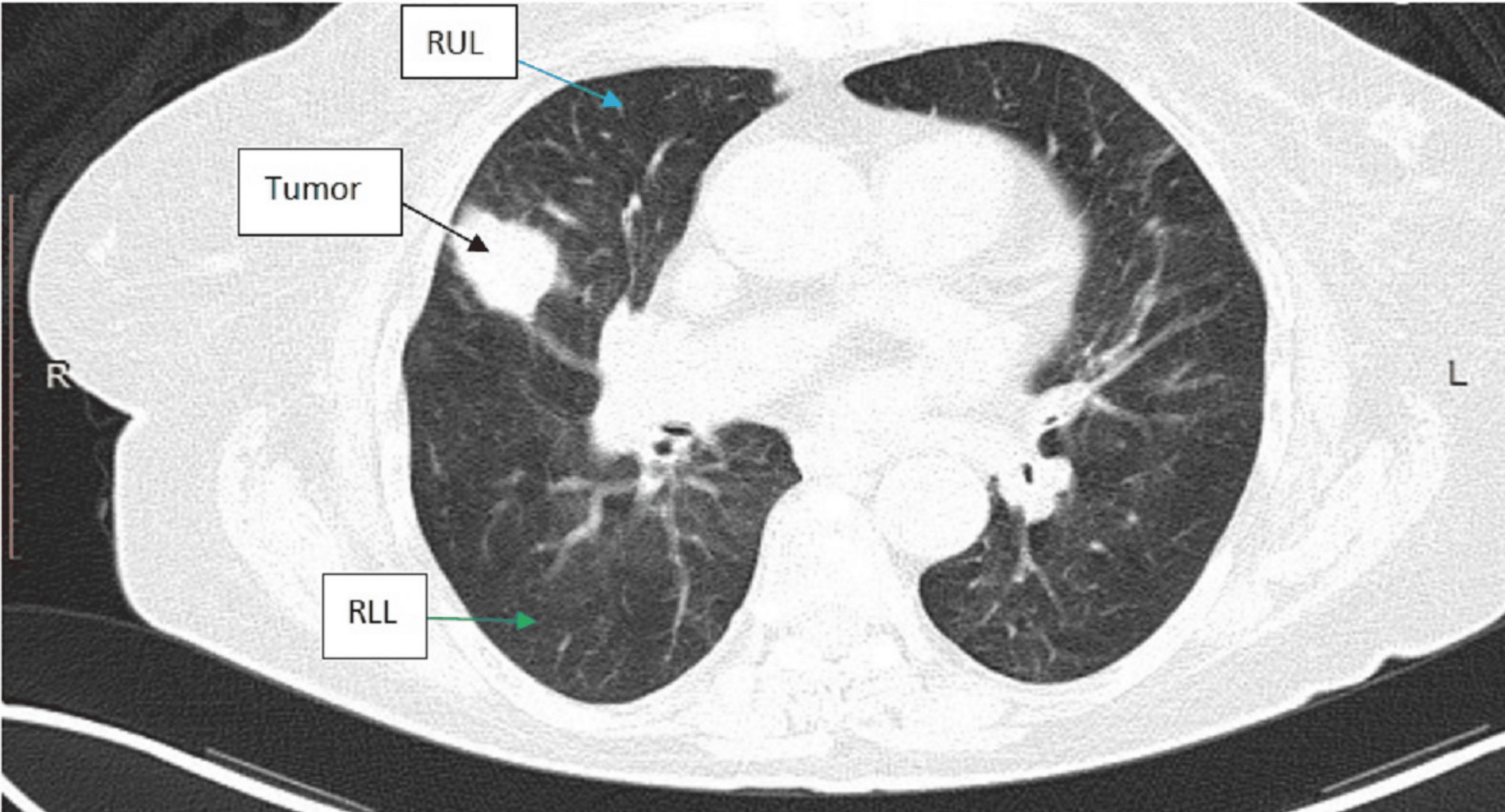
A chest CT revealed a lesion (black arrow) on the posterior segment of the right upper lobe, which was in contact with the minor fissure. RUL: right upper lobe; RLL: right lower lobe
